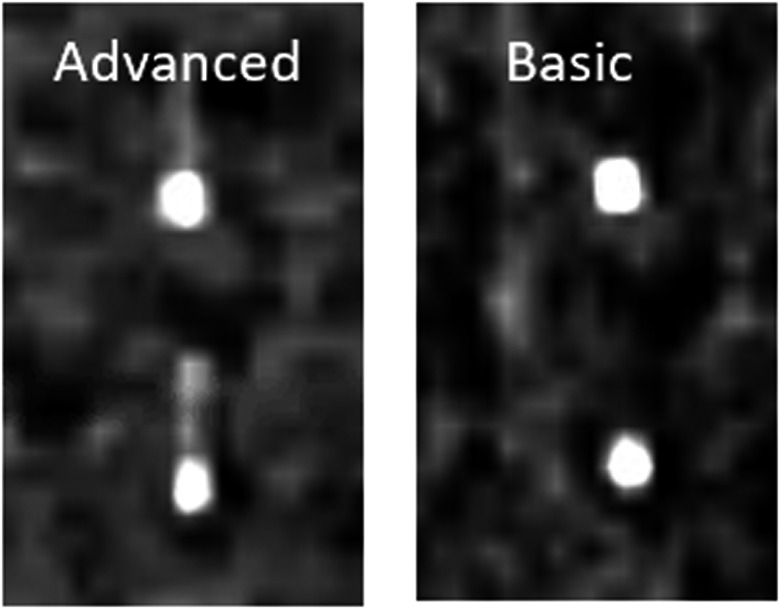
Coronal slices from the 4DCBCT 50% phase bin image of two fiducials placed 2 cm apart in a lung insert reconstructed using the advanced 4D and basic 4D algorithms.
